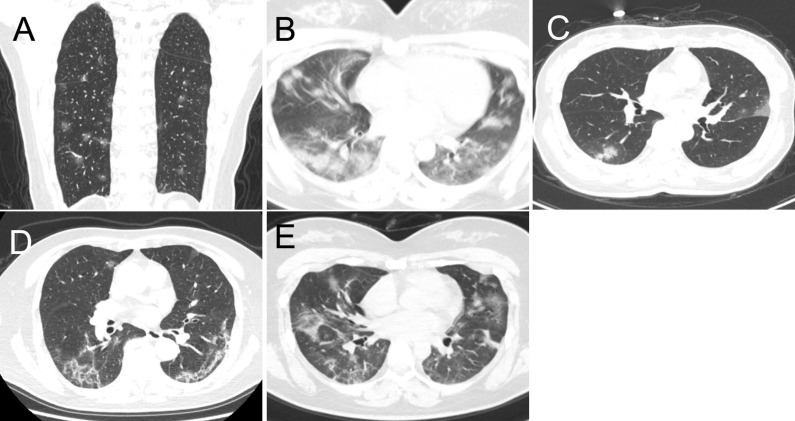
The predominant CT patterns of mild COVID-19 pneumonia. A. GGO. B. Consolidation. C. GGO and consolidation. D. Linear opacity. E. Linear opacity, GGO and consolidation.
